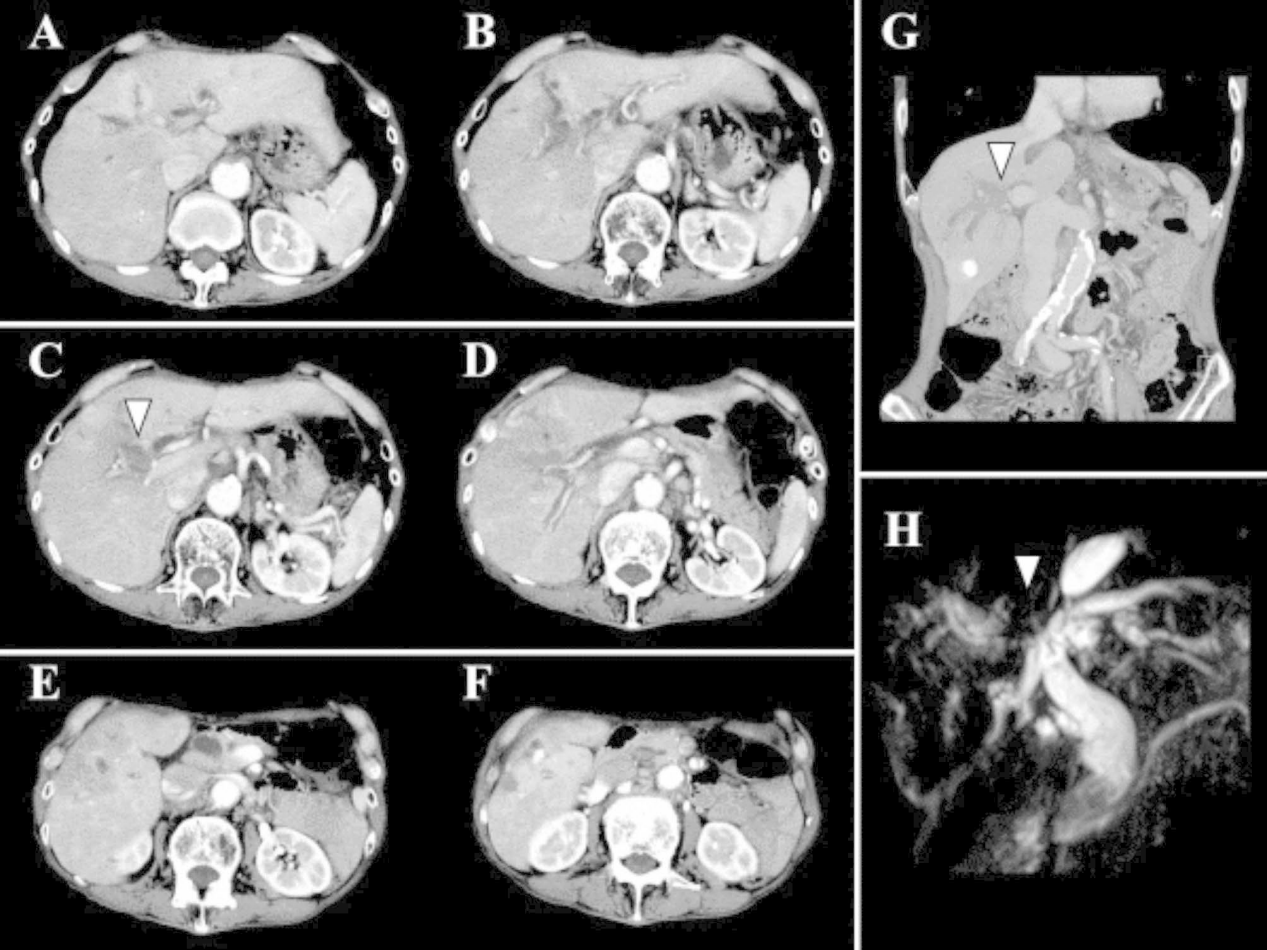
Proton beam therapy for Case 1 and computed tomography images during the follow-up period. (A-C) Dose distribution. The iso-dose lines represent 10–95% of the prescription dose from outside to inside. (D-I) Computed tomography images taken during follow-up 20 months from the start of proton beam therapy did not show any viable lesions and revealed the improvement of dilated bile duct in the liver parenchyma in early phase (D-E) and equilibrium phase (G-I).
